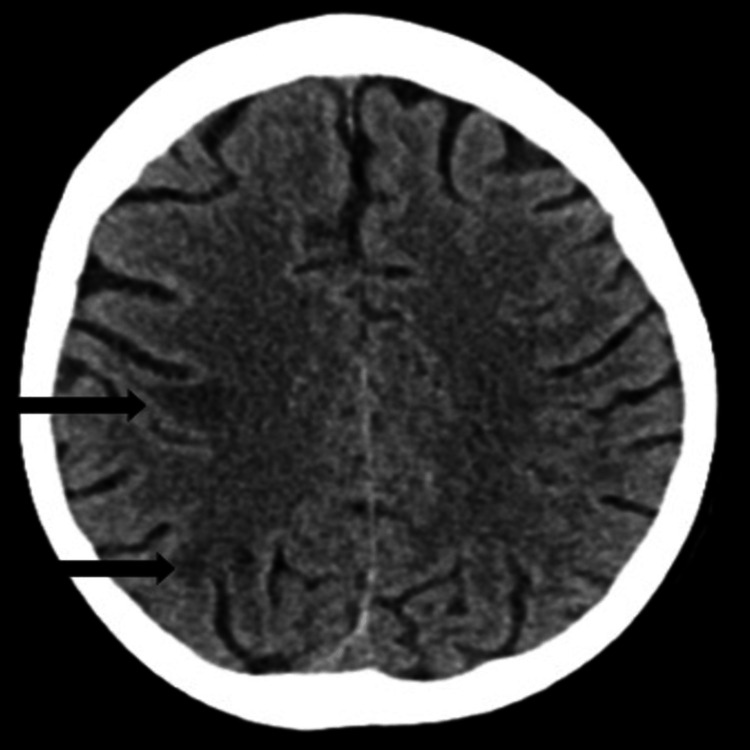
Non-contrast CT head (axial plane) revealing radiological features suspicious for posterior reversible encephalopathy syndrome (PRES).
